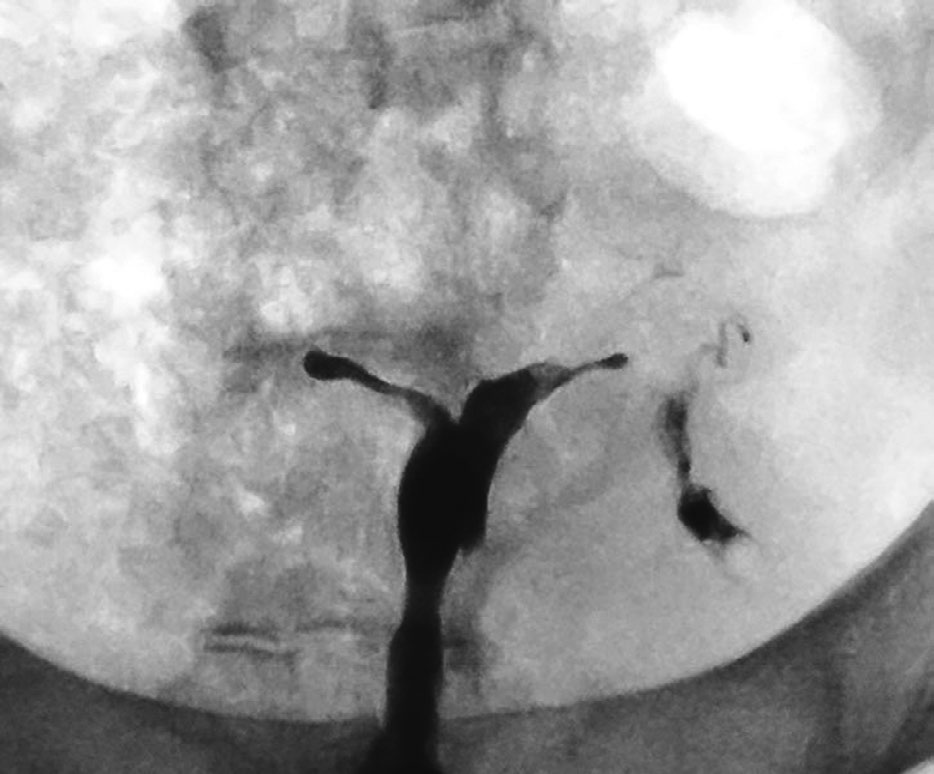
Xray image showing the imprint of a levonorgestrel intra‐uterine device, which had been placed after synecholysis at a different institution, several weeks after removal of the device.
